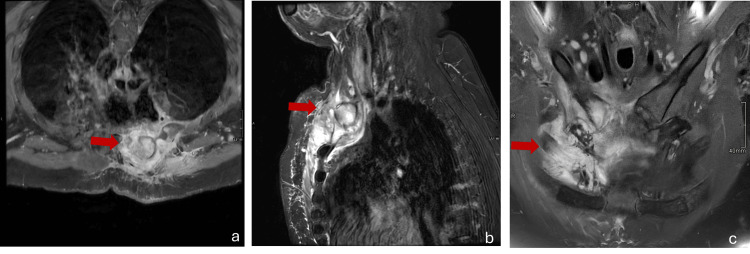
T2-weighted fat-saturated MRI of the right shoulder (a) Axial view, (b) sagittal view, and (c) coronal view Arrows indicate areas of osteomyelitis with associated cellulitis: 7x7 cm hyperintense area of inflammation in the axial plane (a) and 6.5 cm in the anteroposterior dimension on the sagittal plane (b) extending from the right pectoralis muscle through the costochondral junction of the first rib into the right internal mammary space.
